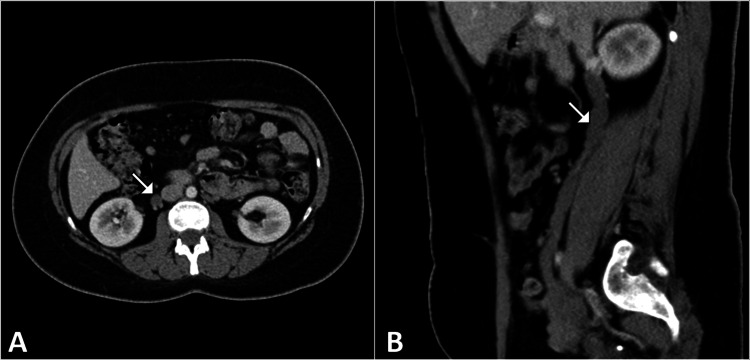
CT images demonstrating right ovarian vein dilatation causing ureteral compression Axial (A) and sagittal (B) contrast-enhanced CT images of the abdomen show a tortuous, dilated right ovarian vein (arrow) originating from the right renal vein and coursing anterior to the right ureter. The ureter is compressed between the dilated vein and the psoas muscle, resulting in upstream hydronephrosis.
